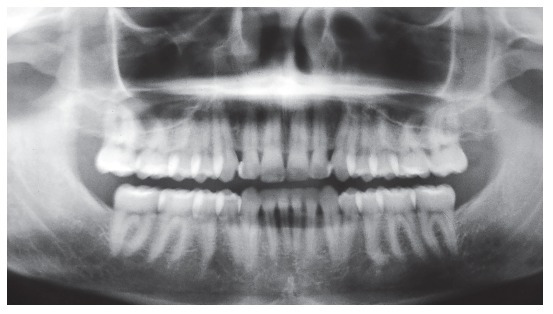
Follow-up panoramic radiograph eight years after treatment completion.
